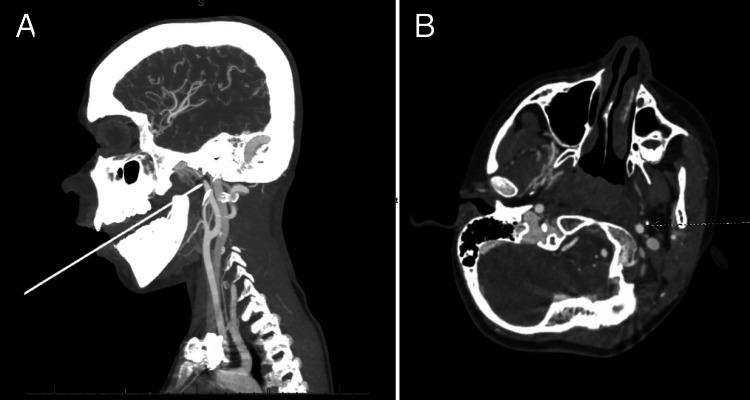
CT angiography demonstrating penetrating oropharyngeal trauma with close proximity to the internal carotid artery (A) Sagittal view of the head and neck showing the penetrating trajectory of the pencil entering the oral cavity and penetrating through the left oropharynx tissue, passing through the medial pterygoid, and continuing into the posterior aspect of the parapharyngeal fat space. (B) Axial view demonstrating the pencil (indicated by the dotted arrow) ending 0.9 mm anterolateral to the internal carotid artery without evidence of vascular extravasation or dissection. CT: computed tomography
